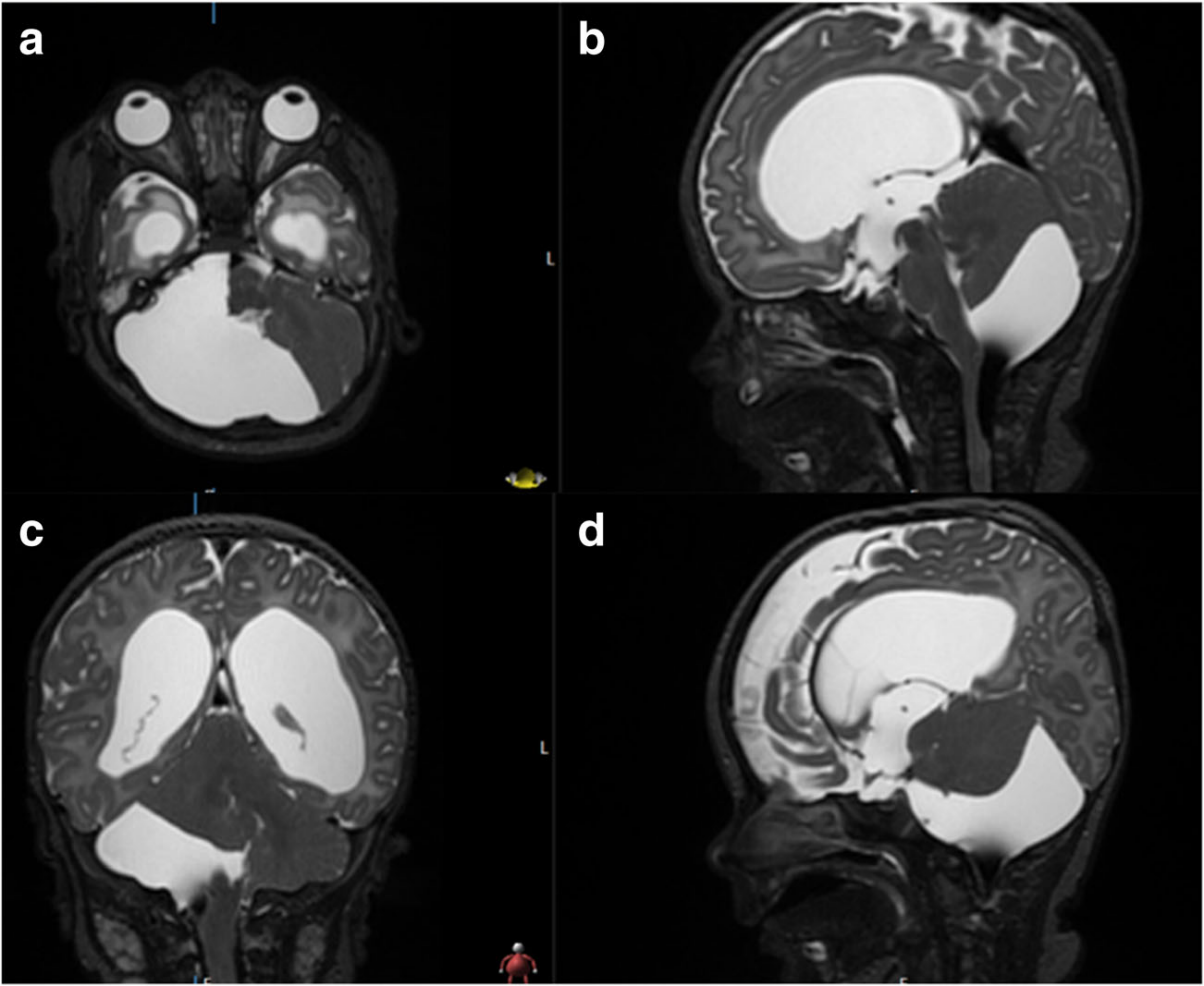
T2-weighted MRI demonstrating hydrocephalus and a posterior fossa AC on initial presentation; axial a; midline sagittal b; coronal c; paramedian sagittal d
